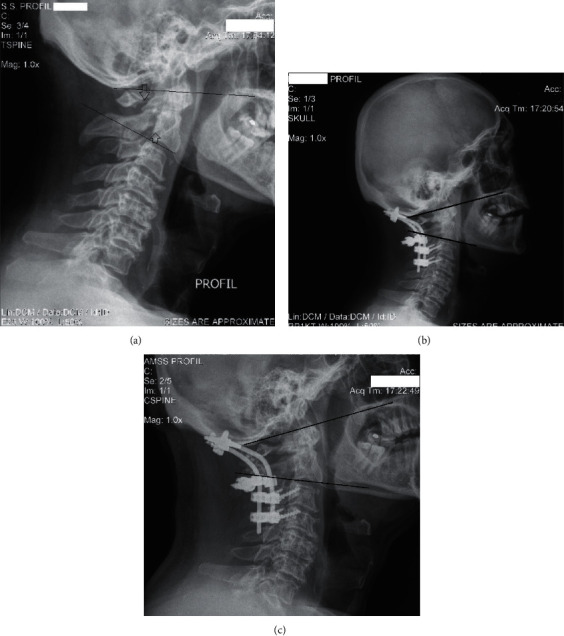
(a) Lateral roentgenogram of a 61-year-old man with C2 Levine IIb traumatic spondylolisthesis with >5 mm diastasis (lower arrow) and comminuted Jefferson fracture (upper arrow). The degeneration in the upper most cervical spine is evident. (b) Postoperative lateral roentgenogram following surgery showing reduction of the injury and POCF. There is an improved lordotic C0-C2 angle compared to the preoperative angle in (a). (c) Follow-up observation 36 months postoperatively showing a complete spinal fusion and a lordotic C0-C2 angle.
